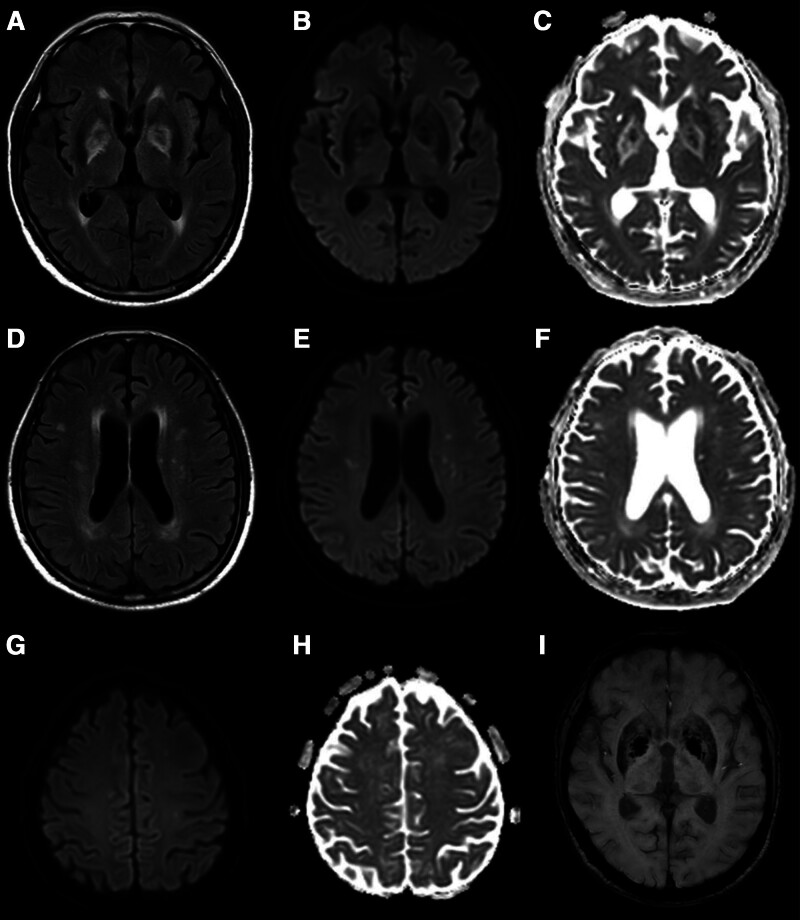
A 76-year-old man with COVID-19 and pneumonia was transferred to our hospital on day 9 after the onset of pneumonia symptoms due to worsening respiratory status and altered mental state. Because his drowsy mental state persisted, a brain MRI was performed 14 days after transfer. (A) The MRI revealed symmetrical high signal intensity lesions in the bilateral globus pallidus on a fluid attenuation inversion recovery image. (B, C) These lesions did not show restricted diffusion on DWI and the apparent diffusion coefficient map. (D–H) In addition, multiple punctate lesions with high DWI signal intensity were noted in the bilateral cerebral white matter. The distribution of these lesions did not correspond to typical watershed territories but was more consistent with an embolic infarction pattern. (I) There were no hemorrhagic changes observed in the globus pallidus on susceptibility-weighted imaging, apart from dark signal intensity due to iron deposition. COVID-19 = coronavirus disease 2019, DWI = diffusion-weighted imaging, MRI = magnetic resonance imaging.
